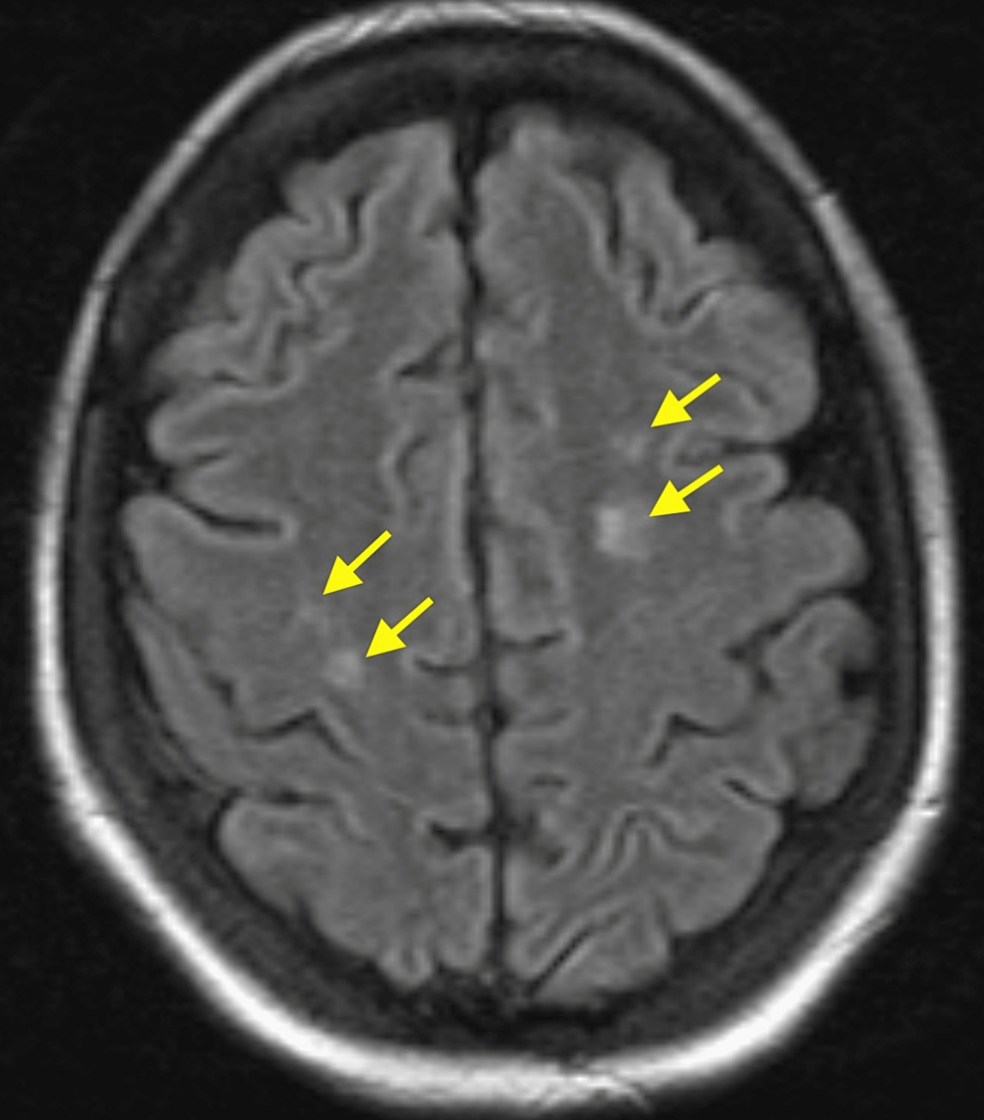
FLAIR sequence on brain MRI depicting multiple hyperintense signal foci (yellow arrows).
